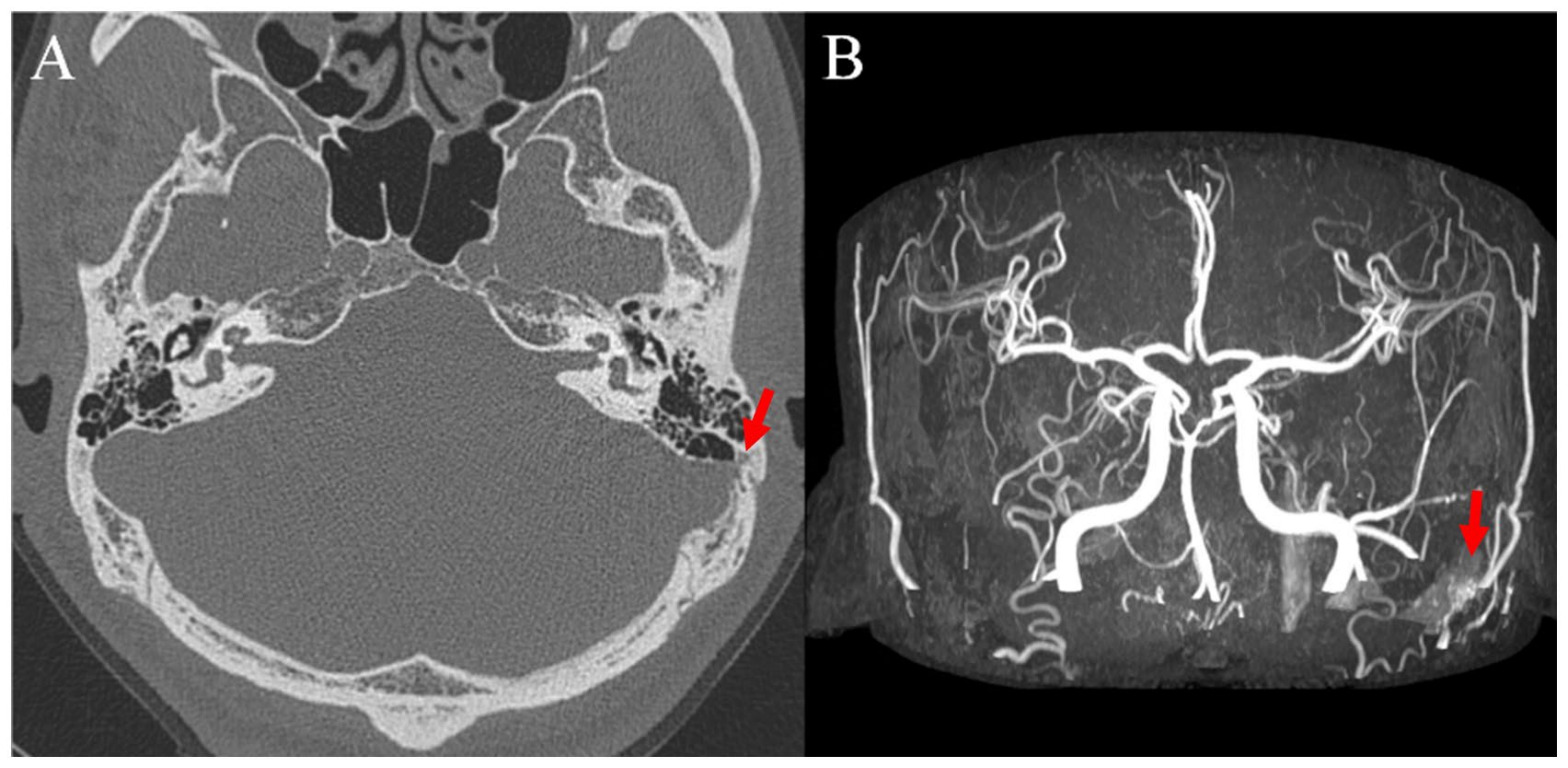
(A) Preoperative axial temporal bone computed tomography image showing left sigmoid sinus dehiscence and (B) postoperative brain magnetic resonance image with angiography image showing left dural arteriovenous fistula.
